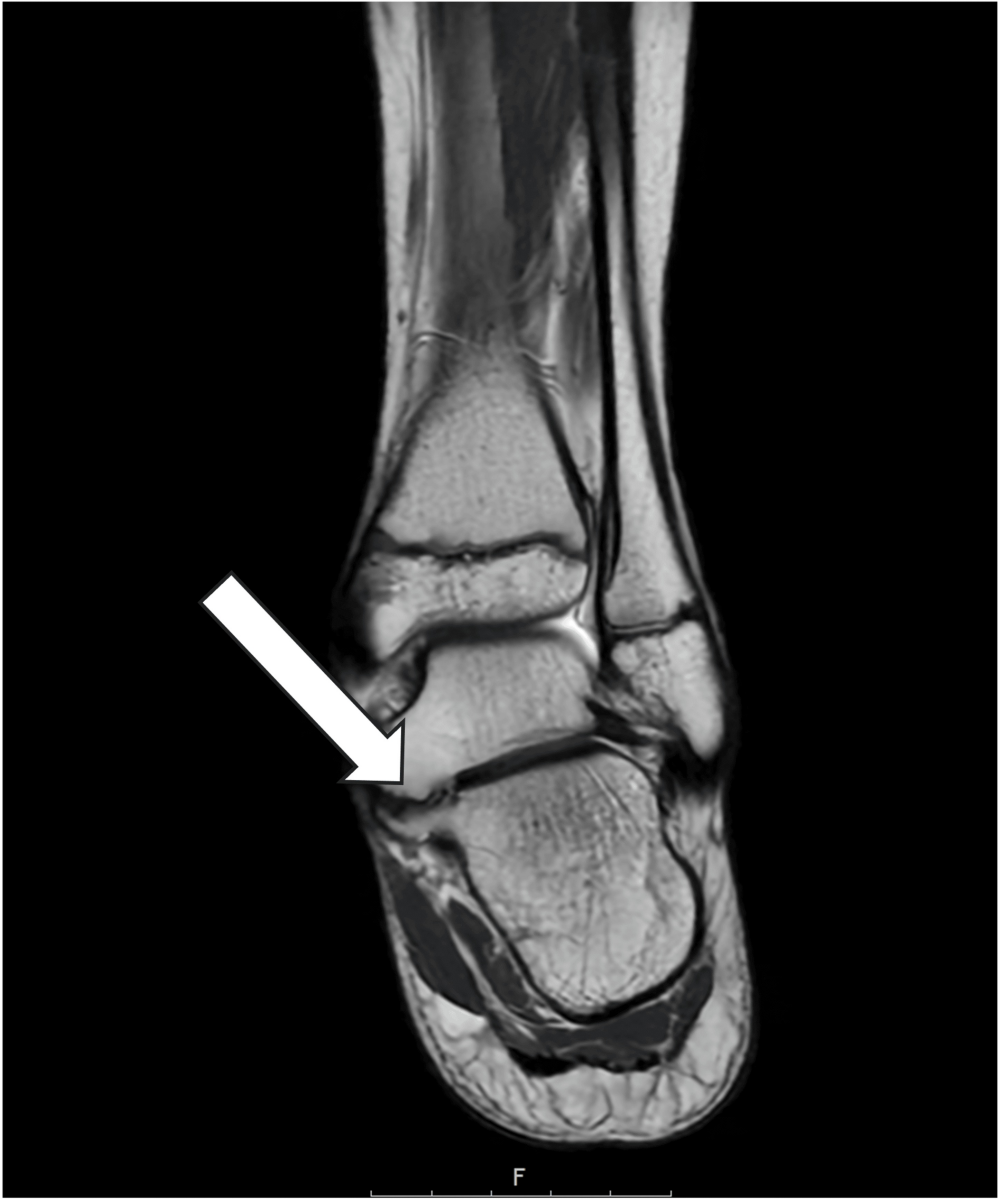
Preoperative coronal T2-weighted MRI confirming the non-osseous talocalcaneal (TC) coalition, shown with the white arrow.
